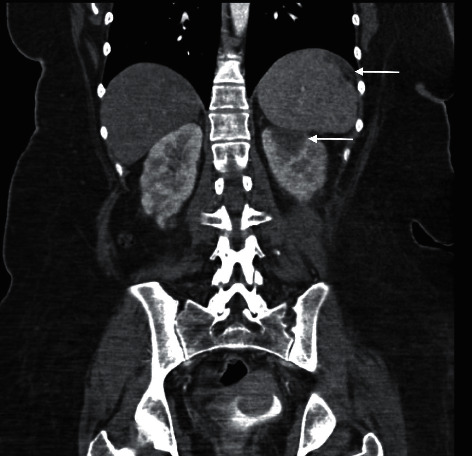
CT angiogram of the abdomen (arterial phase) showing left superior renal infarction and splenic infarction.
